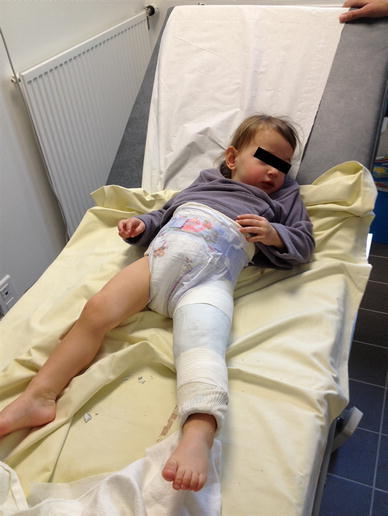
Photograph of a 2-year-old girl after temporary spica cast immobilization before MRI for great trochanter chronic pain
